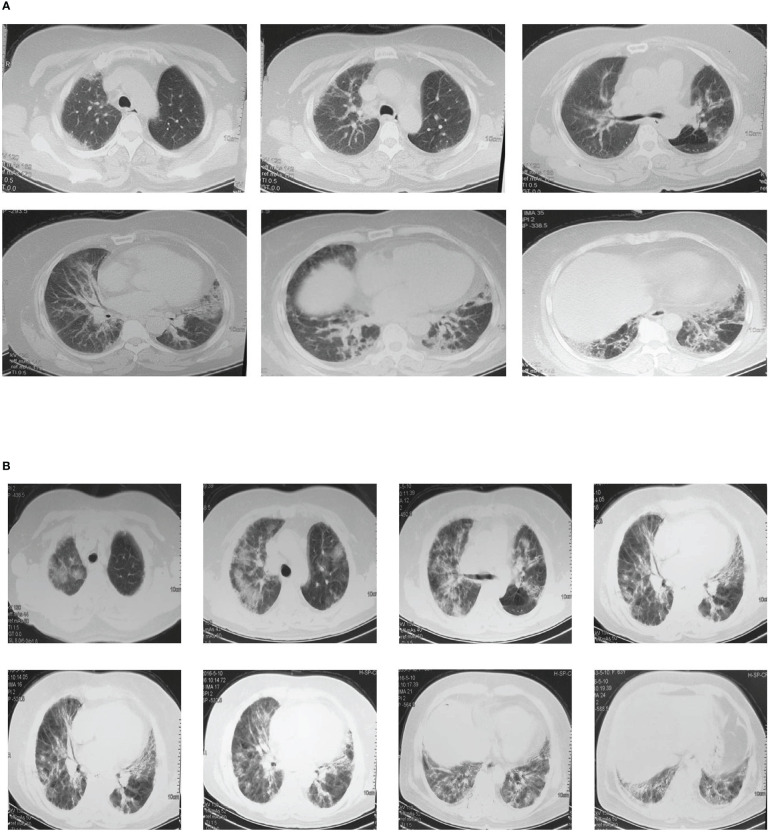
Chest CT images of the proband. (A) Multiple ground-glass and grid shadows distributing around the lungs and under the pleura were found in both lungs In December 2015. (B) Multiple patchy shadows, grid shadows and cable shadows were diffusely distributed in both lungs, and some of the shadows fused into large areas in May 2016.
